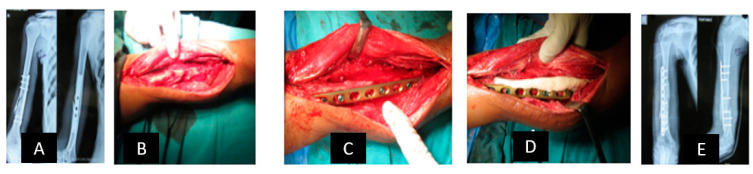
A 19-year-old patient was referred with swelling induration and sinus discharge 6 months after operative management of a humerus shaft fracture. (A) Preoperative X-ray: AP and lateral view. (B) Intraoperative photographs showing purulent discharge. (C) Through debridement excision of sequestrated bone stabilization with a locking compression plate (LCP) using locking screws only. (D) Filling of the bone defect with antibiotic-impregnated bone cement. (E) AP and lateral views after stage one.
